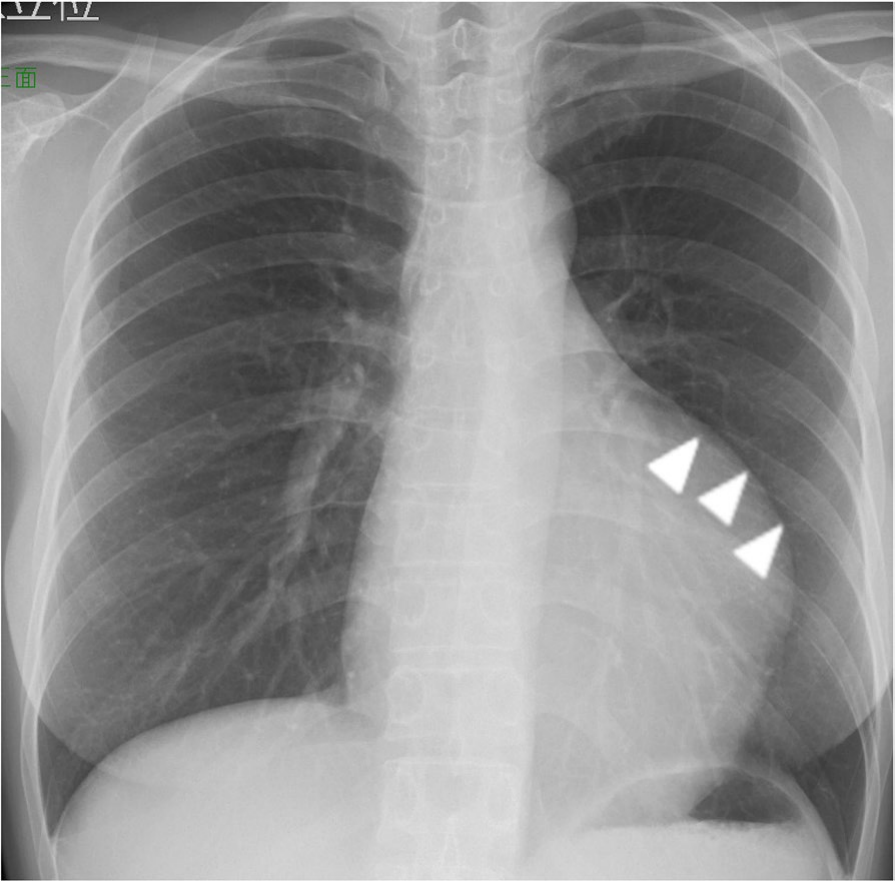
Chest X-ray showing protrusion of the left upper cardiac margin (white arrowhead)
